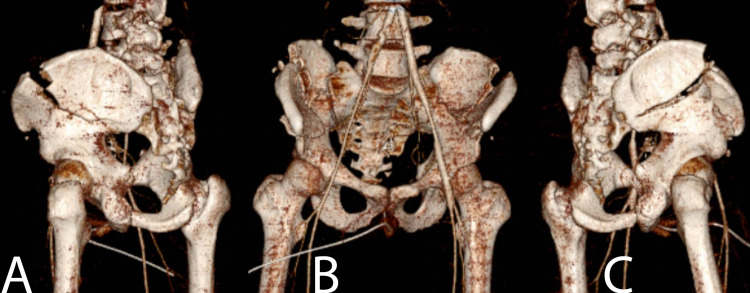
3D reformatted CT images of the pelvis. (A) 3D reformatted CT image demonstrating left crescent fracture as well as iliac wing fracture. (B) 3D reformatted CT image demonstrating bilateral crescent fracture-dislocation as well as bilateral iliac wing fractures. (C) 3D reformatted CT image demonstrating right crescent fracture as well as iliac wing fracture. 3D: three-dimensional; CT: computed tomography
